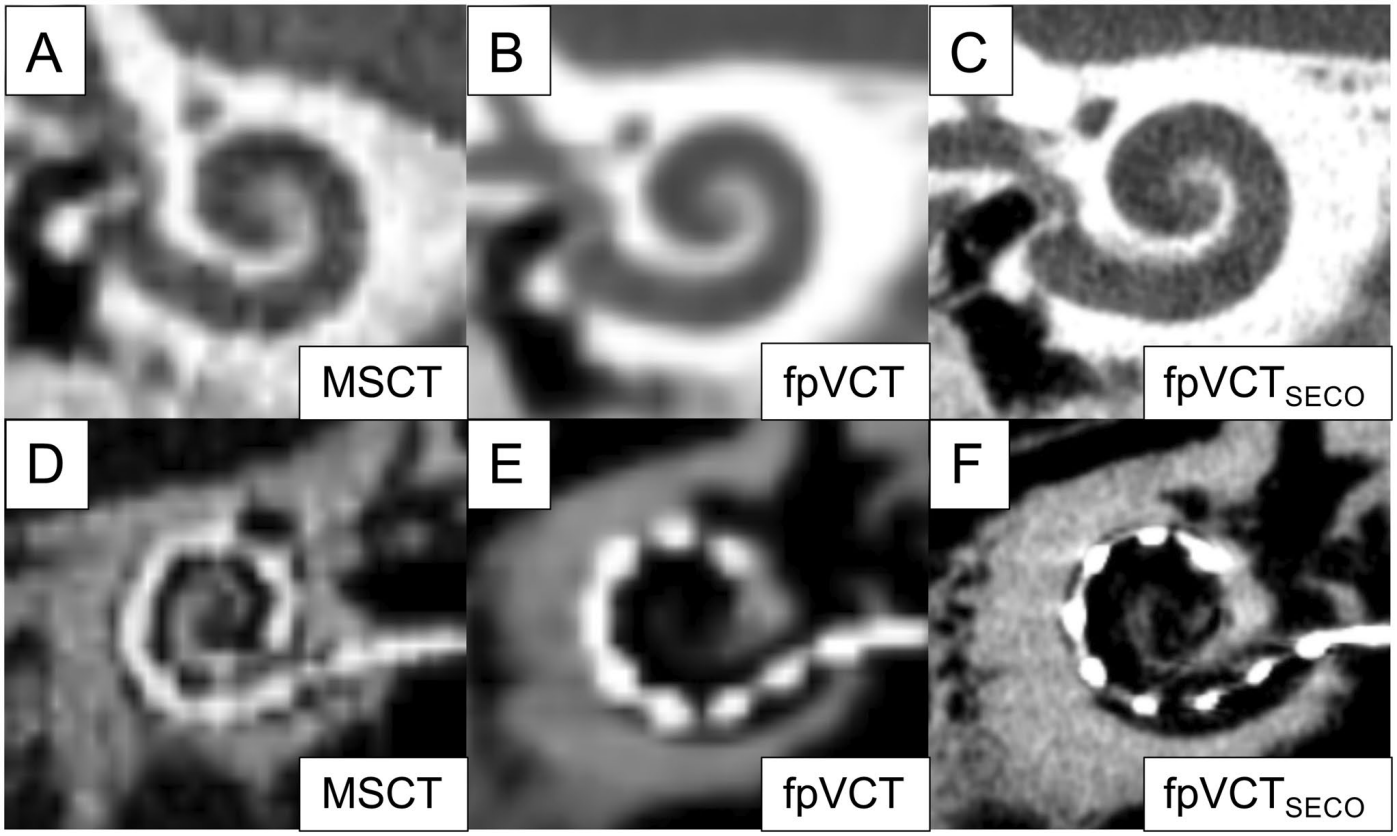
“Cochlear view” of different imaging modalities and settings. Representative image of MSCT (600 µm) is shown in panel (A), display of fpVCT (466 µm) in panel (B). Image of fpVCT_SECO_ (99 µm) is presented in panel (C). Respectively, panels (D–F) demonstrate the “Cochlear View” [31] with an implanted electrode. MSCT: multislice computed tomography, fpVCT: flat-panel volume computer-tomography, fpVCT_SECO_: fpVCT secondary reconstruction
